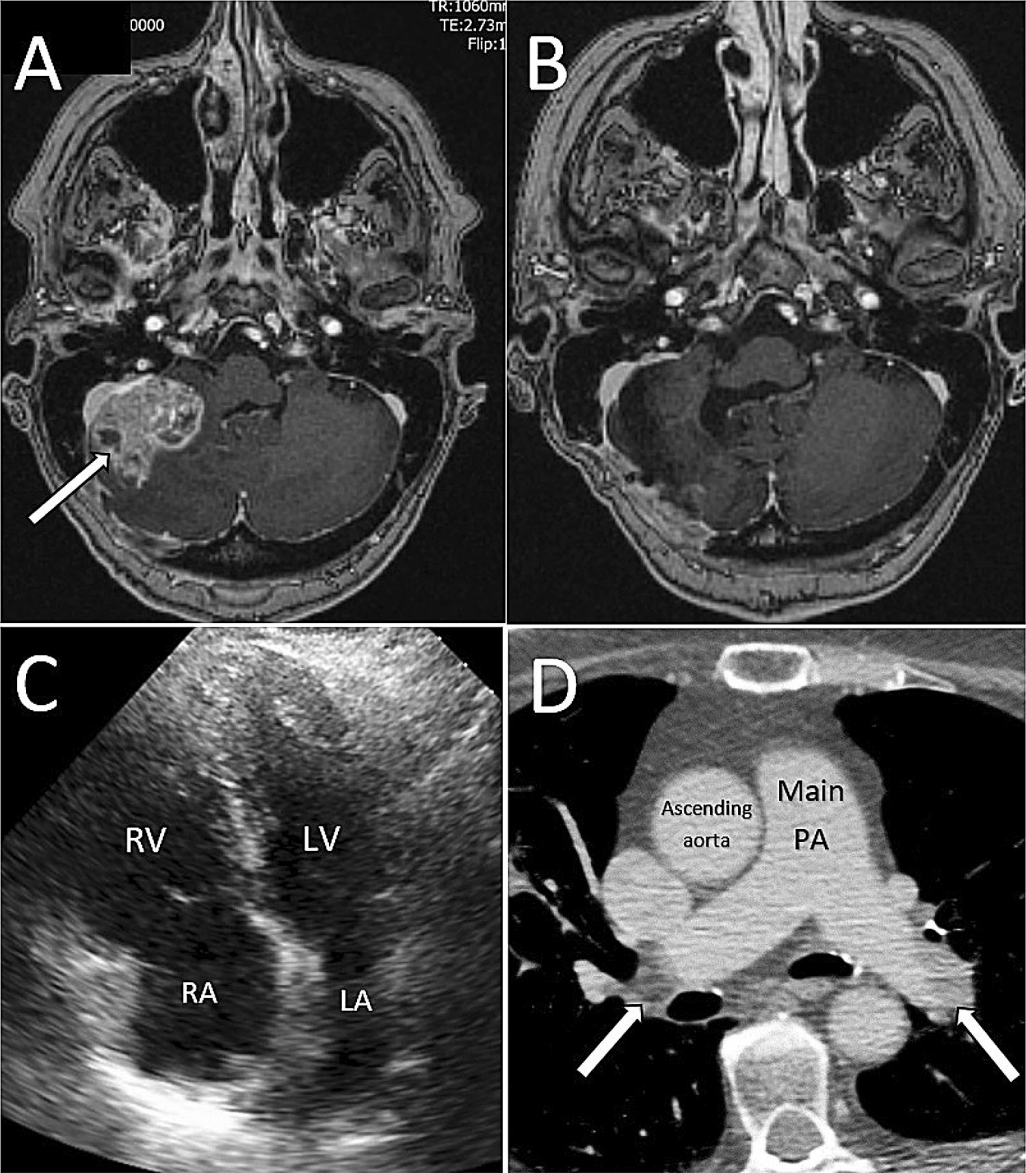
a Right sided solitary cerebellar metastasis pre, and b post-surgery, c Echocardiogram showing dilated right ventricle, d CTPA with gadolinium contrast showing bilateral PE
